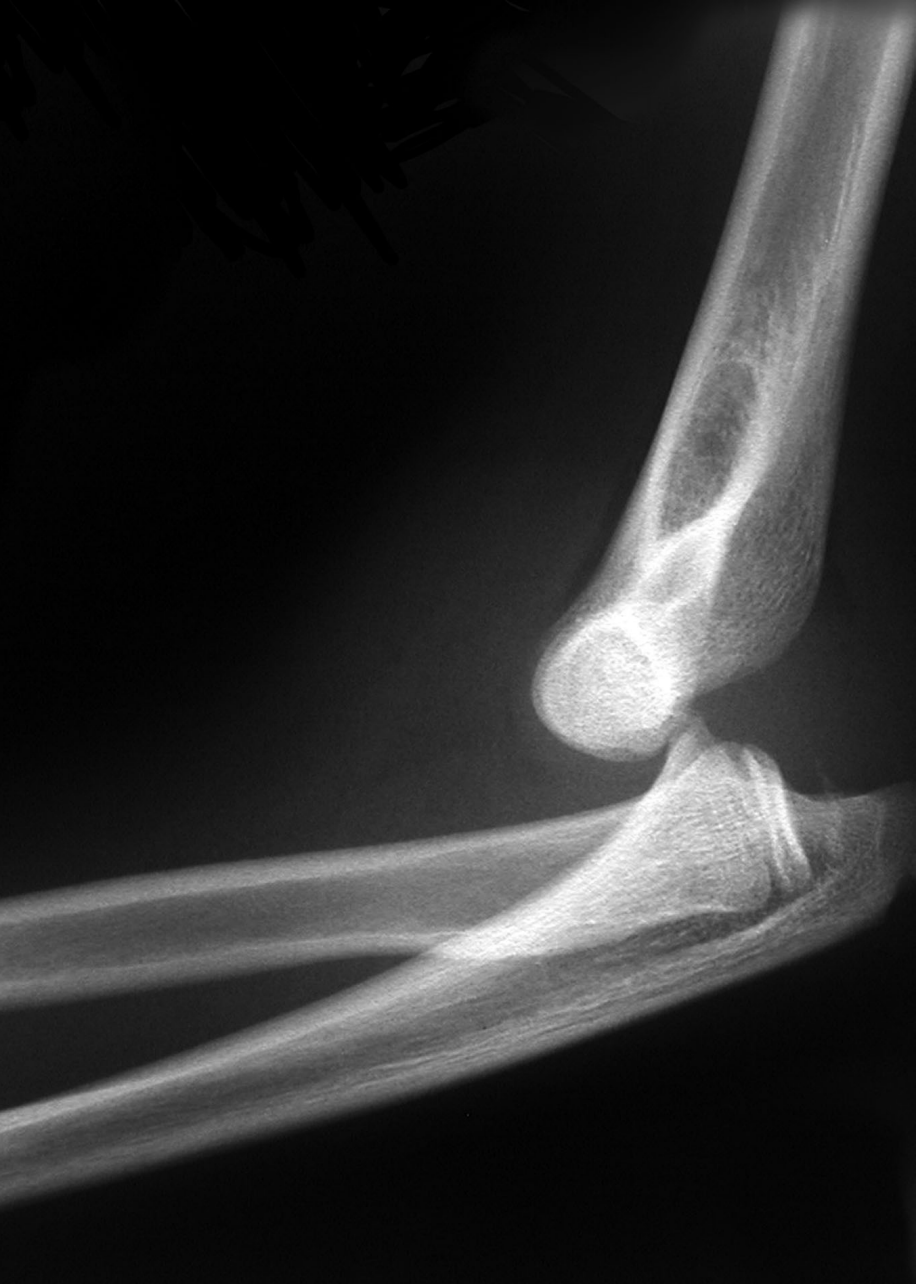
Preoperative lateral radiograph of the elbow revealing elbow dislocation.
